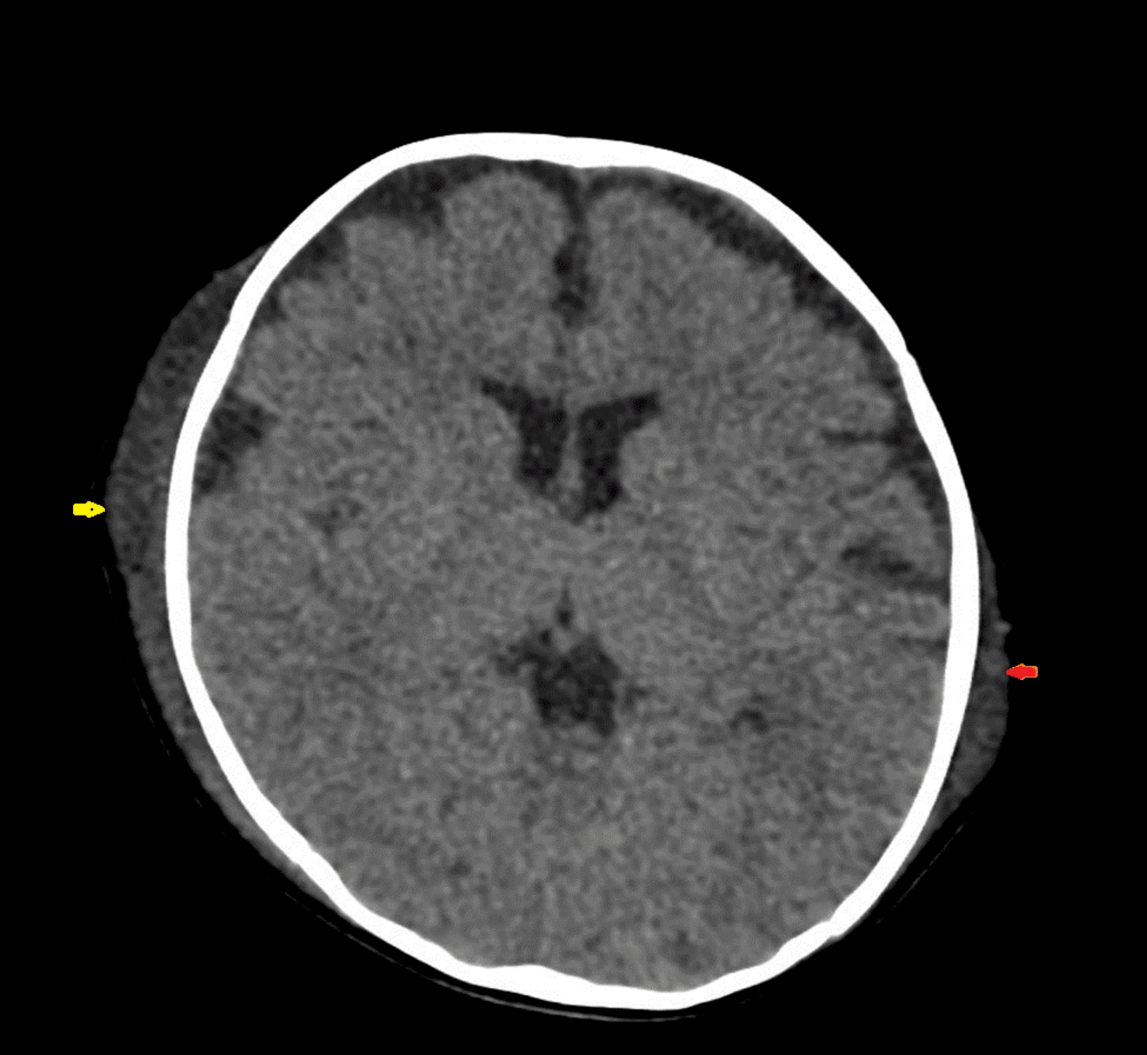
CT of the brain (axial plane) showing right-sided subgaleal hematoma (yellow arrow) with involvement of the opposite side (red arrow).
